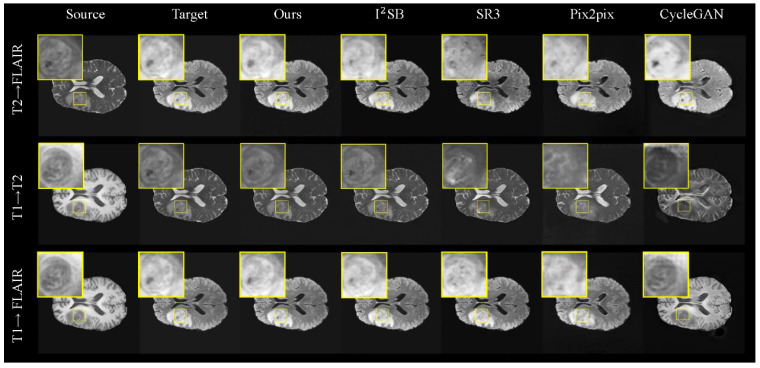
Generated MRI images for various tasks in the BraTS 2021 test set using different generation methods. The yellow boxes highlight the brain tumor region. The FADM significantly outperforms the other methods, displaying excellent texture details and structural accuracy, especially in the tumor area. The generation quality is better for the T2 modality compared to the FLAIR modality.
